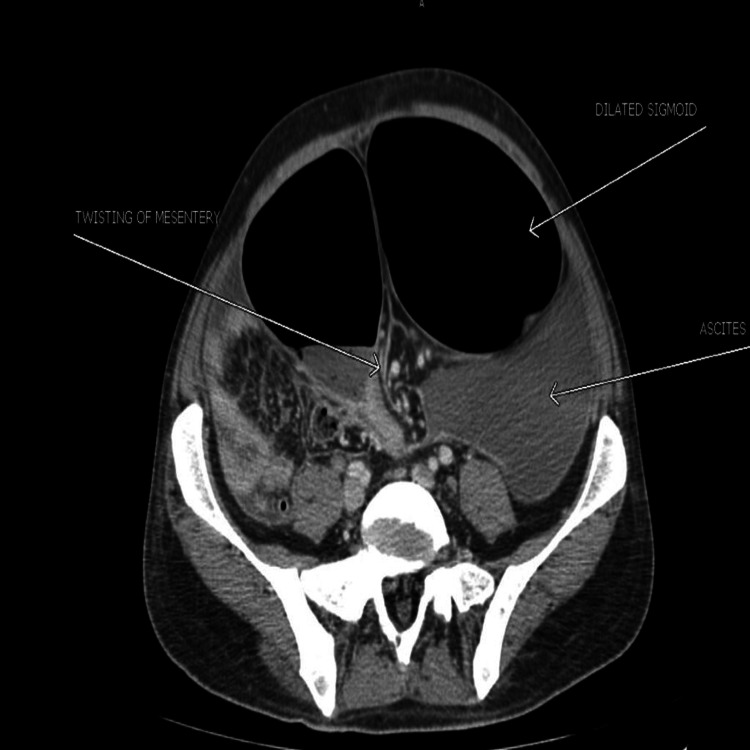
CT scan showing the dilated sigmoid with twisting of the mesentery and gross ascites
